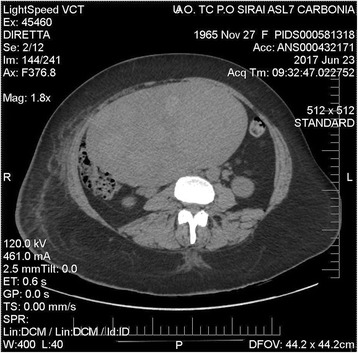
Case 2: axial computed tomography scan of the abdomen showing a huge inhomogenous uterus measuring more than 20 cm occupying entirely the right side of the pelvis
